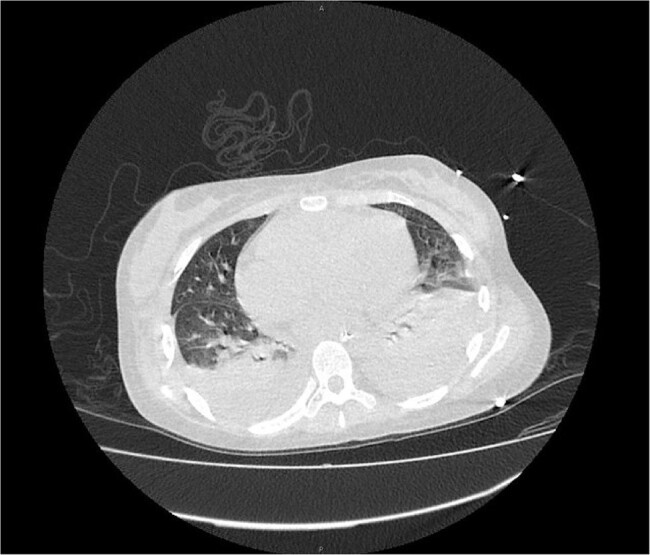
Cross-sectional computed tomography of the lungs in a patient diagnosed with pneumonic tularemia by microbial cell-free DNA (mcfDNA) testing, showing bilateral moderate pleural effusion and right upper and middle lobe pulmonary nodules. This patient also exhibited nonspecific mediastinal and bilateral axillary lymphadenopathy and typhoidal symptoms. In this case, mcfDNA testing identified Francisella tularensis nearly 50 hours before any conventional testing confirmed the diagnosis and prompted changes in antibiotic therapy and infection prevention efforts to begin.
